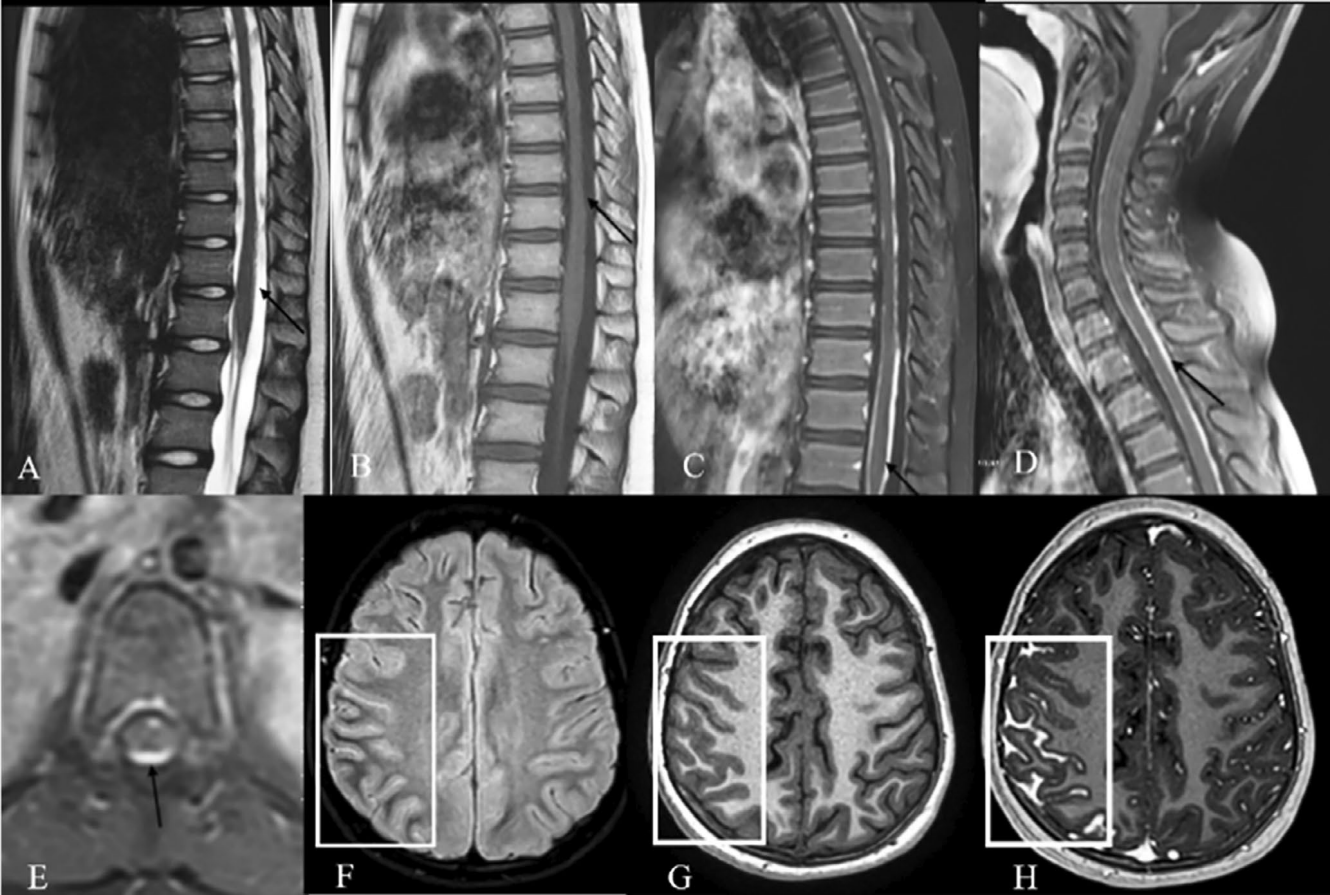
MRI images from the lumbar, thoracic, and cervical spine showing diffuse leptomeningeal. Irregularity and mild thickening (A, sagittal T2‐weighted; B, sagittal non‐contrast T1‐weighted sequences) with significant irregular enhancement on post‐contrast T1‐weighted sequences (C–E, axial views). Brain MRI demonstrated hyperintensity of sulci in the frontoparietal lobes on FLAIR sequence (F), which is obliterated on axial non‐contrast T1 sequence (G) and shows thick enhancement on post‐contrast T1 sequence (H). The involved areas are indicated within the white box.
